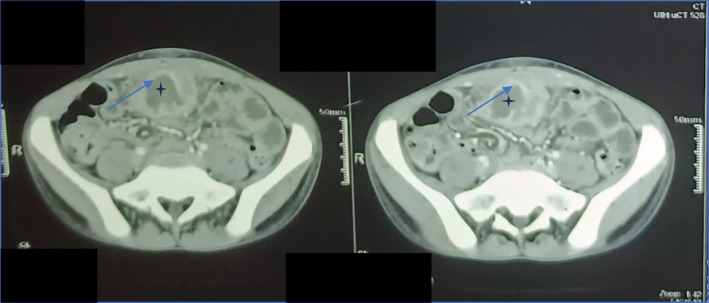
Contrast‐enhanced axial computed tomography (CT) of the abdomen. The axial view reveals a large, 7.1‐cm complex inflammatory mass (phlegmon) located in the midline of the anterior lower abdominal wall. The lesion demonstrates a thick, peripherally enhancing wall (arrow) and a central, low‐density necrotic core (asterisk). Significant inflammatory fat stranding surrounds the mass, effacing the tissue planes and confirming dense adherence to adjacent loops of the distal ileum.
